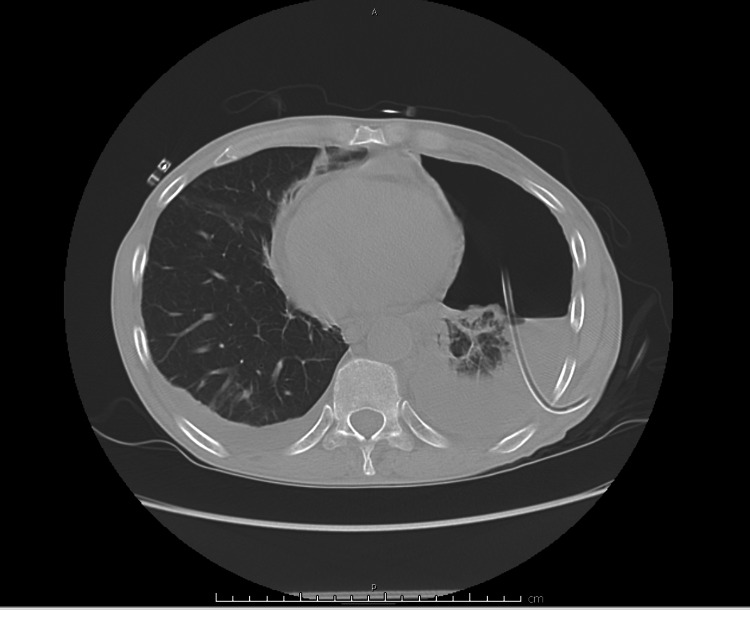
CT Chest post PleurX catheter placement
